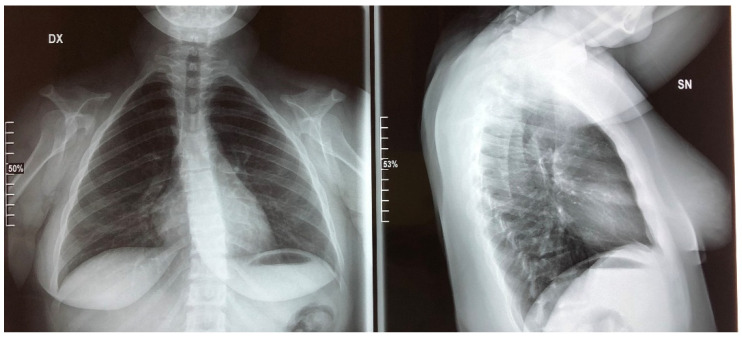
Thorax X-ray in 2021 showing a narrow and bell shape thorax.
